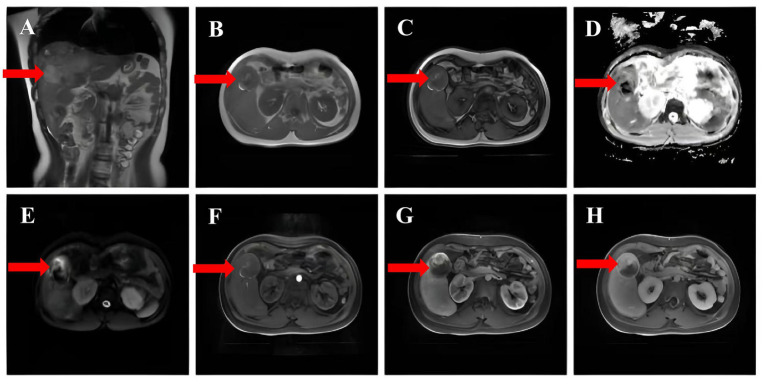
(A) Fast echo spin (FSE) showing mixed hypointense signals. (B,C) No significant signal reduction in the same inverse phase was observed. (D,E) DWI and ADC revealed no significant diffusion restriction. (F,G,H) Contrast-enhanced MRI scans with delayed phase enhancement.
